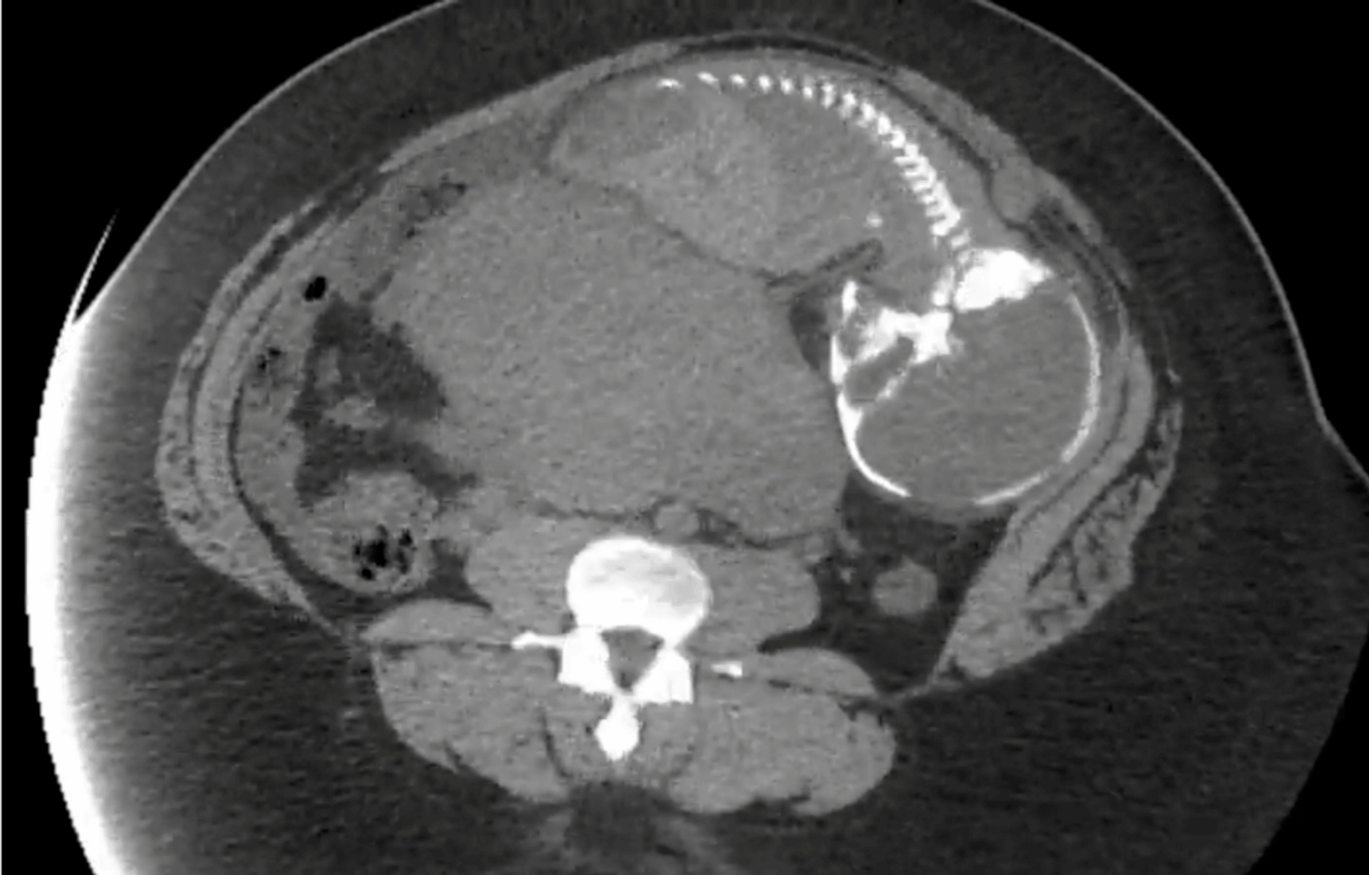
Noncontrasted CT scan showing an intraperitoneal fetus
